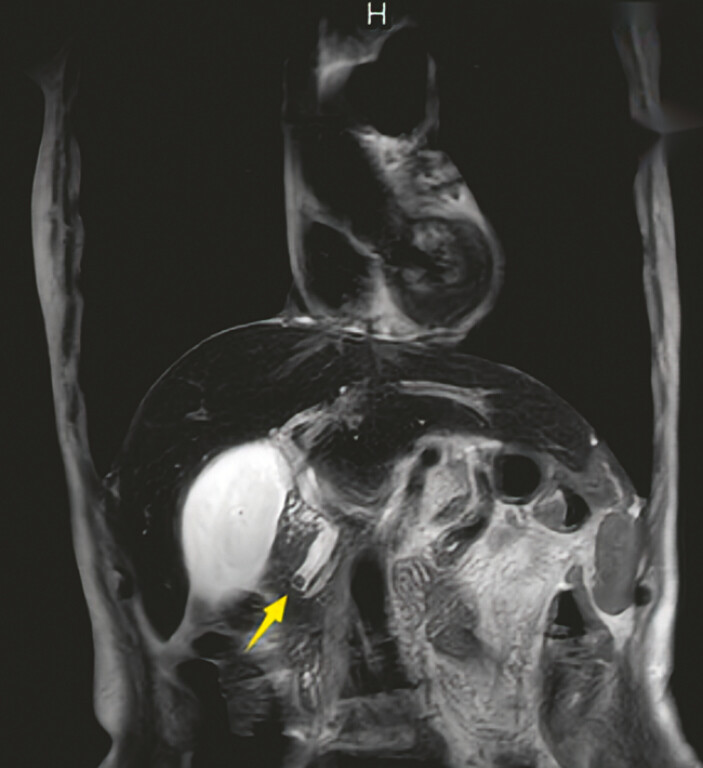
Magnetic resonance imaging revealed a stone in the common bile duct and mild dilation of the upstream duct (indicated by the yellow arrow).
